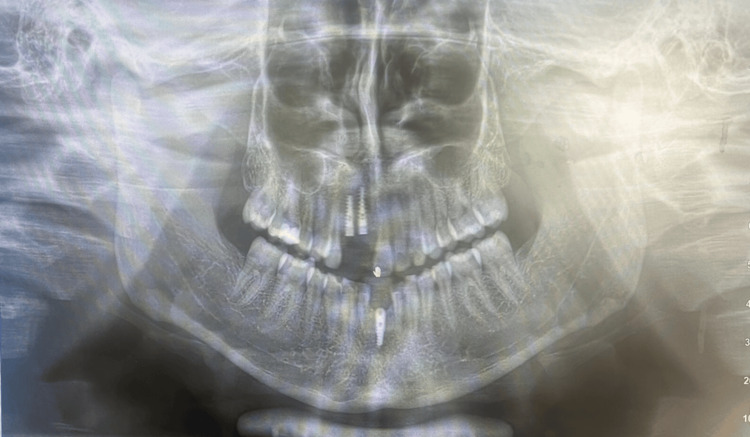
Post-implant placement orthopantomogram (OPG).
